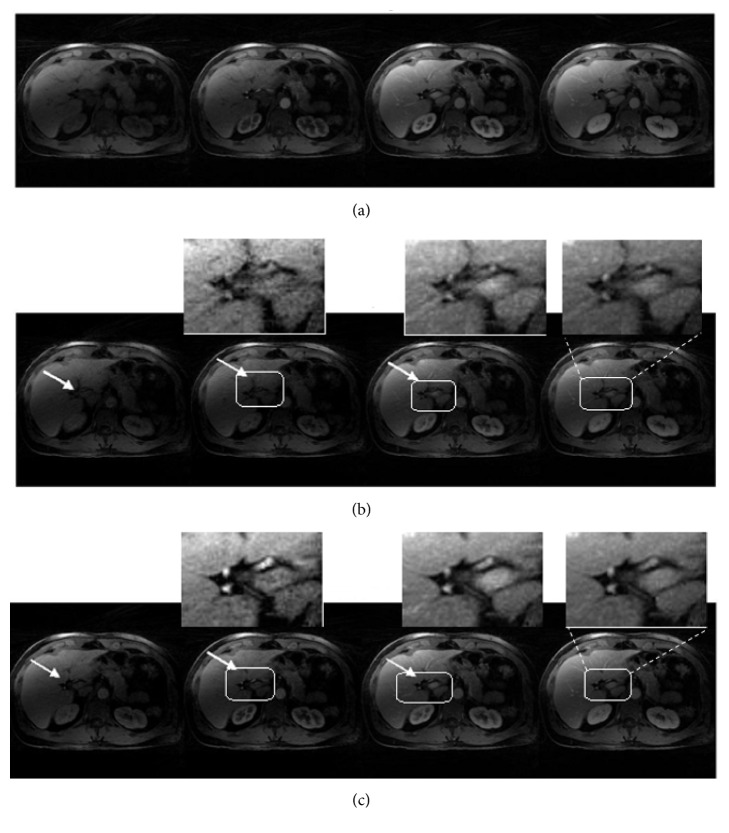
Qualitative comparison: reference images (a) CL+S images (before binning) (b) and HL+S images (after binning) (c) for liver DCE MRI. Without binning recovery suffered from respiratory motion blurring effects. In contrast, the proposed method enabled improved reconstruction of all phases, better capture of the arterial phases, and higher vessel clarity and sharpness. These effects can be observed in magnified views.
